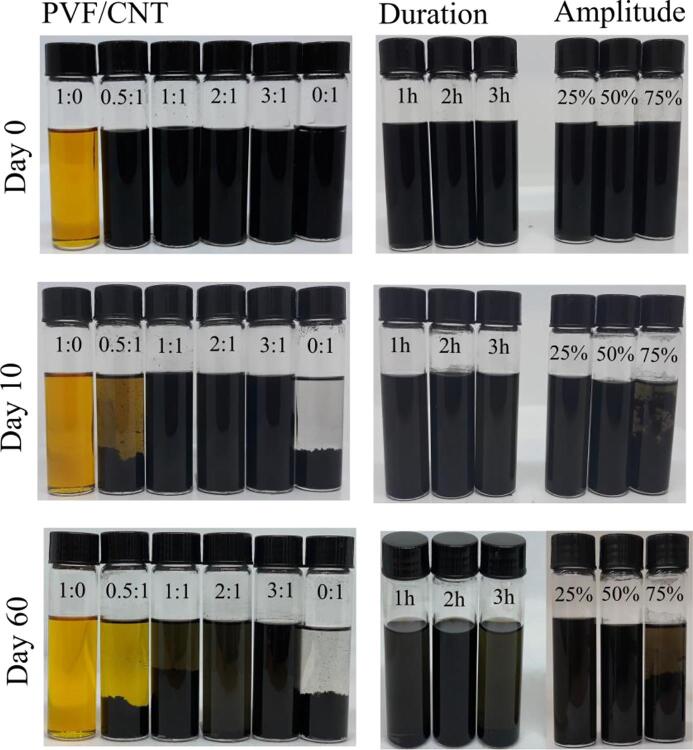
Effect of PVF/CNT, sonication duration, and ultrasonic amplitude on the sedimentation behaviors of the prepared electrode solutions. Photographs of the PVF/CNT dispersions in chloroform on day 0, day 10, and day 60.
